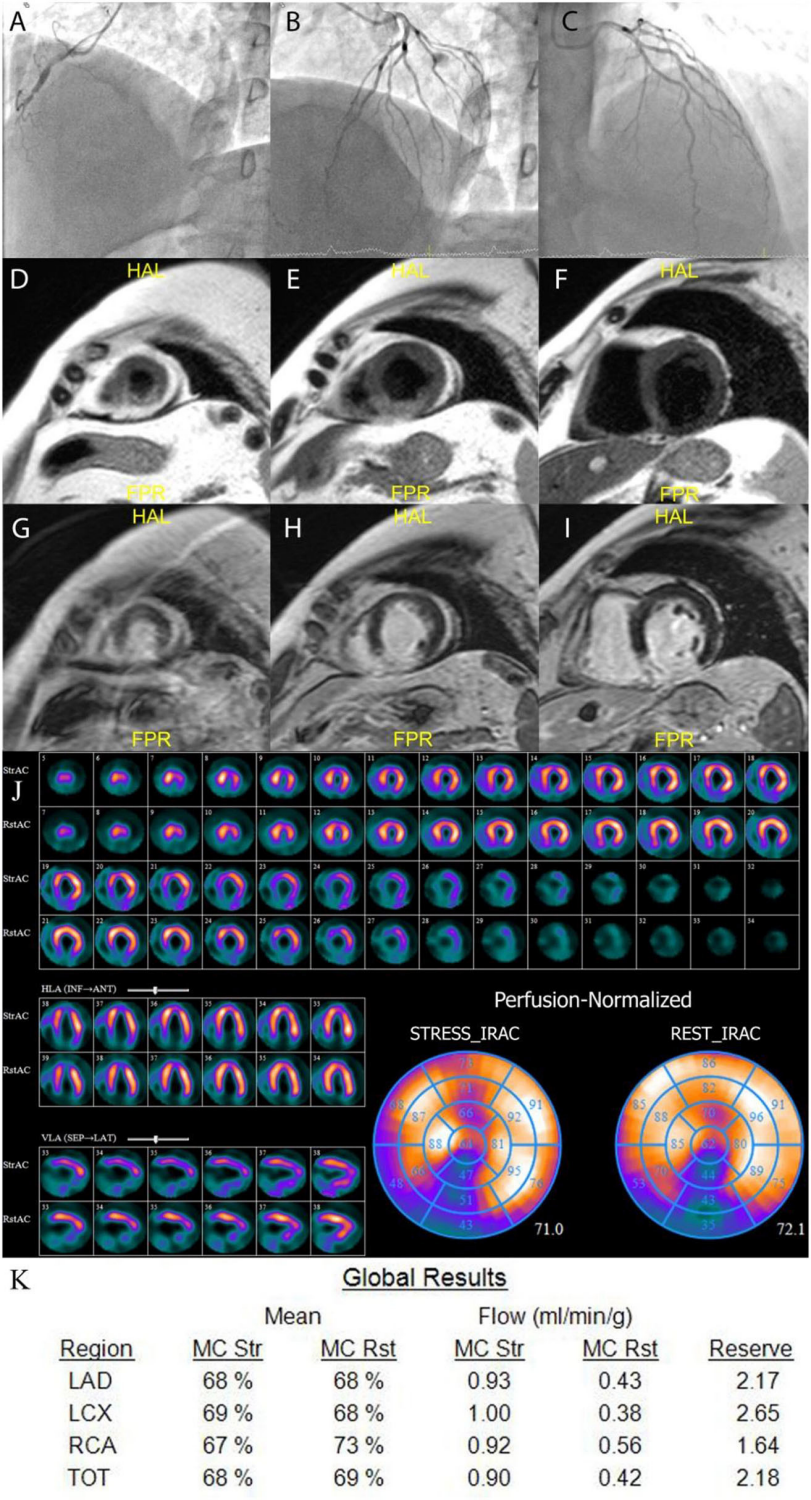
Example of MICAD patient. A 55 years old male with RCA occlusion (A) and no CAD in LAD and LCx (B,C). CMR results: T2-weighted images (D–F) show transmural edema in the posterior and posterior-septal segments; late gadolinium enhancement images (G–I) show high signal intensity in the same regions indicating necrosis in the posterior and posterior-septal segments. The qualitative MPS (J) shows severe fixed perfusion defect in posterior wall and small reversible defect in mid and basal anterior wall. The quantitative CZT SPECT results (K) show abnormal MFR in RCA territory. LAD, left anterior descending artery; LCx, left circumflex artery; RCA, right coronary artery; TOT, total value; MC, motion correction; mL/min/g, milliliters per minute per gram; Rst, rest; Str, stress.
